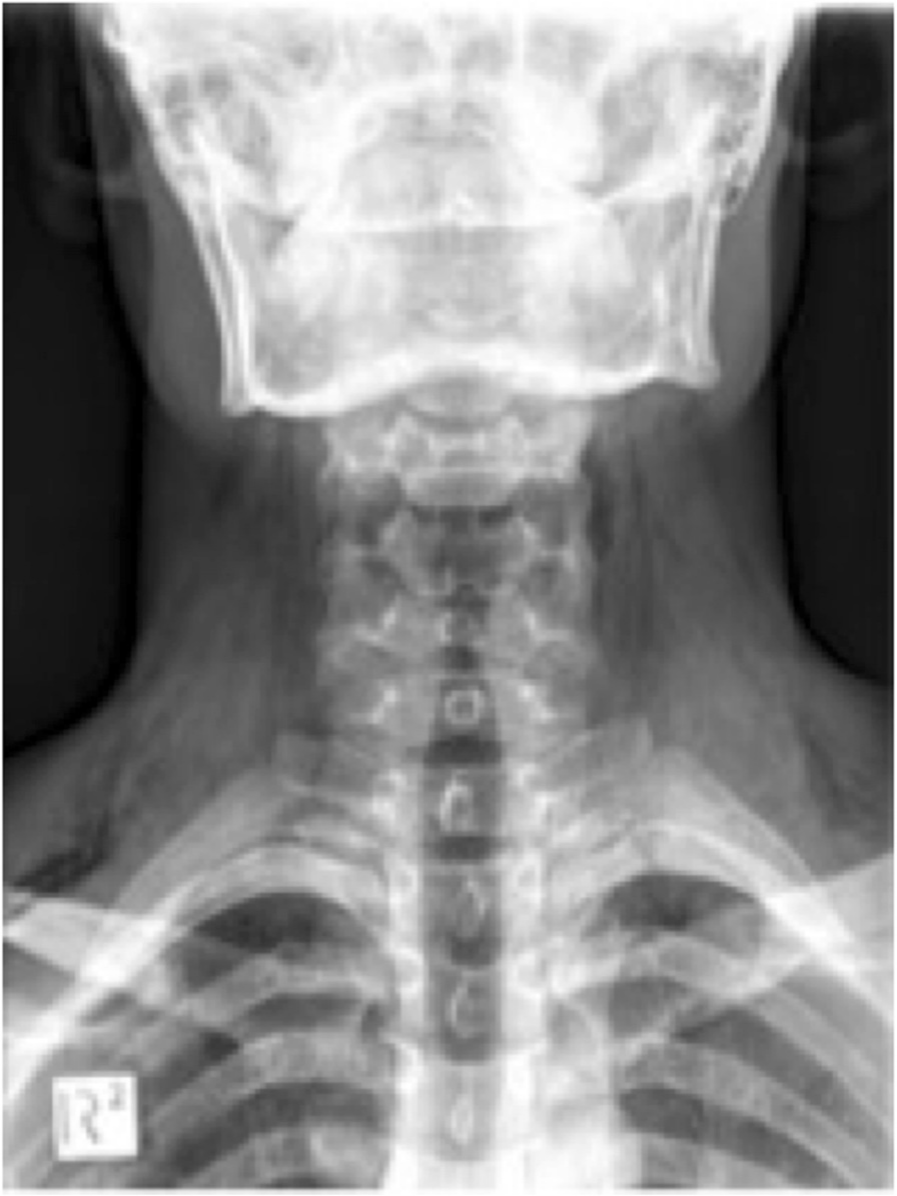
Plain radiograph of the neck showing extensive subcutaneous emphysema
